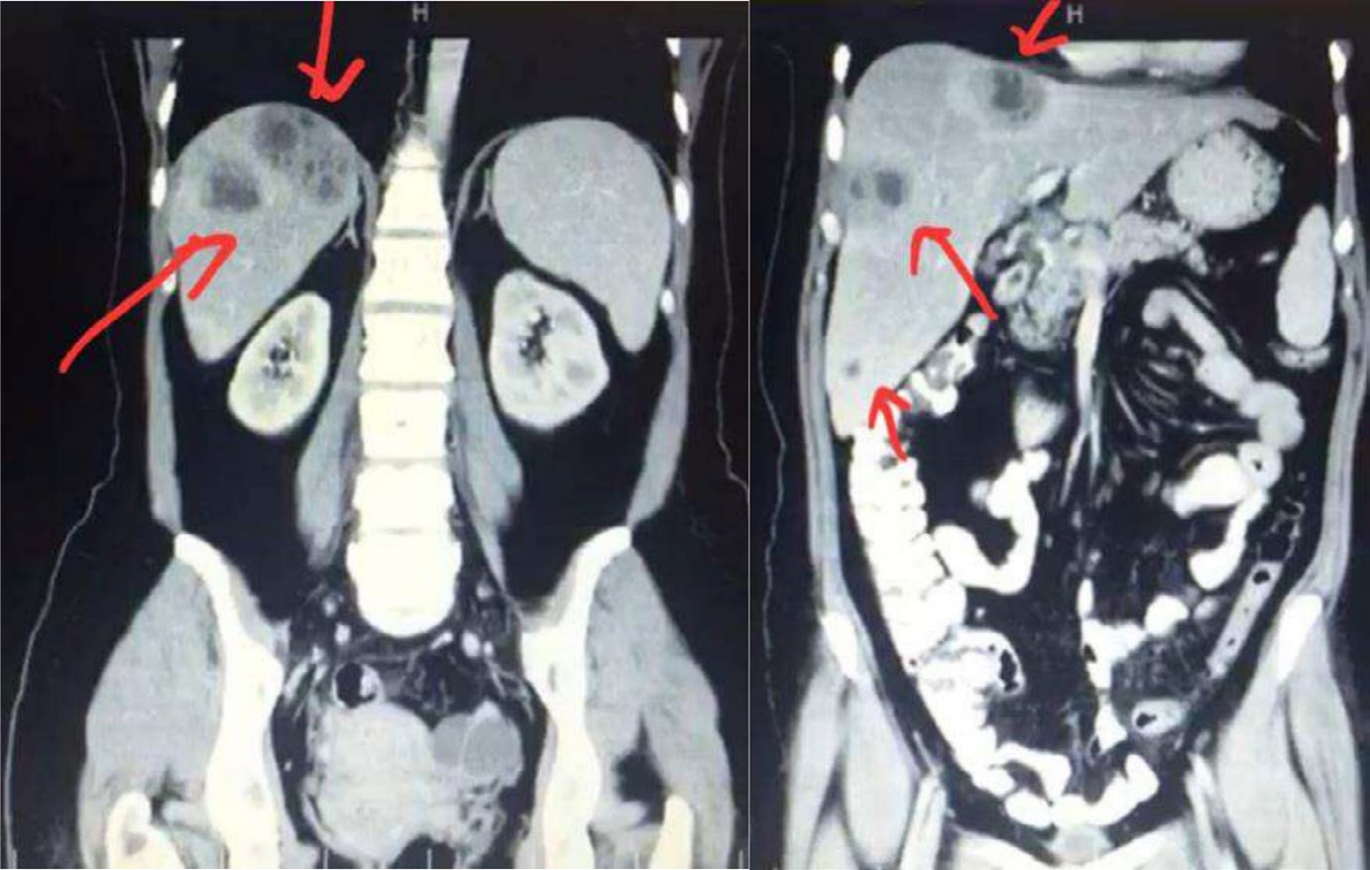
CT scan of abdomen 4 months before admission showing multiple abscesses in the liver with no pleural effusion.
